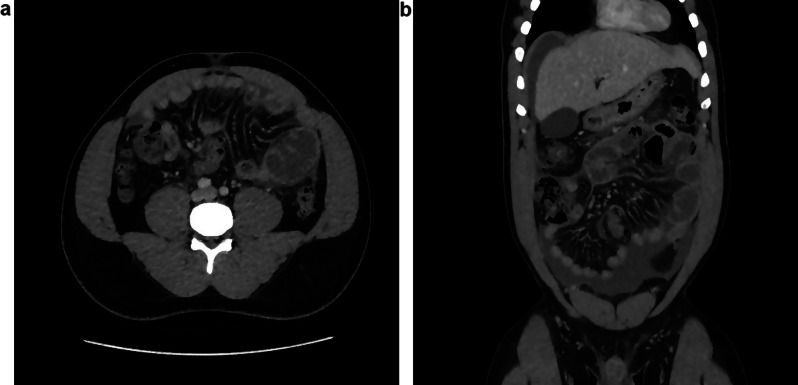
(a) and (b): CT scan showing jejunal distension, increased intra-abdominal effusion with sign of peritonitis
